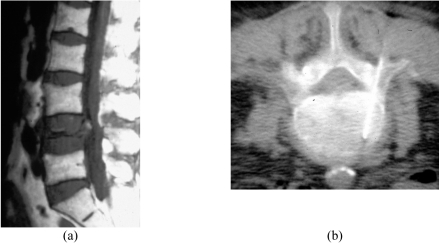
Severe L4 vertebral body compression in a 77-year-old woman. (a) Sagittal SE T1-W MR image shows severe compression of the L4 vertebral body. (b) Axial CT fluoroscopic image taken with the patient lying prone shows the Ostycut needle inserted into the right side of the L4 vertebral body via the transpedicular route.
